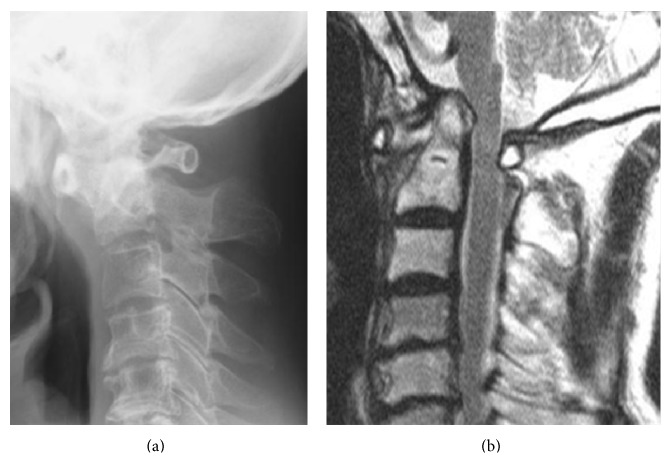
Images six months after the treatment of intravenous antibiotics and a halo immobilization showing vertical subluxation on the odontoideum and disappearance of the abscess. (a) Lateral view. (b) Sagittal T2-weighted image.
